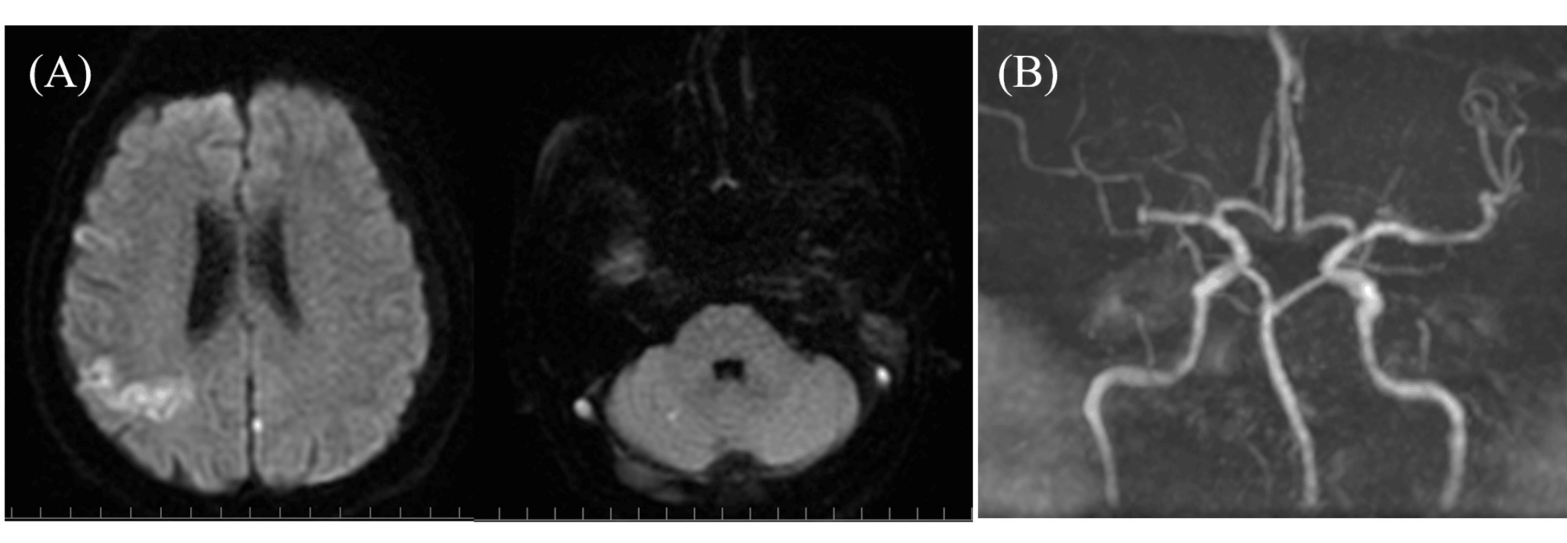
Head magnetic resonance image on admission: (A) Axial diffusion-weighted image shows multiple cerebral infarctions; (B) Magnetic resonance angiography shows no significant major arterial stenosis.
